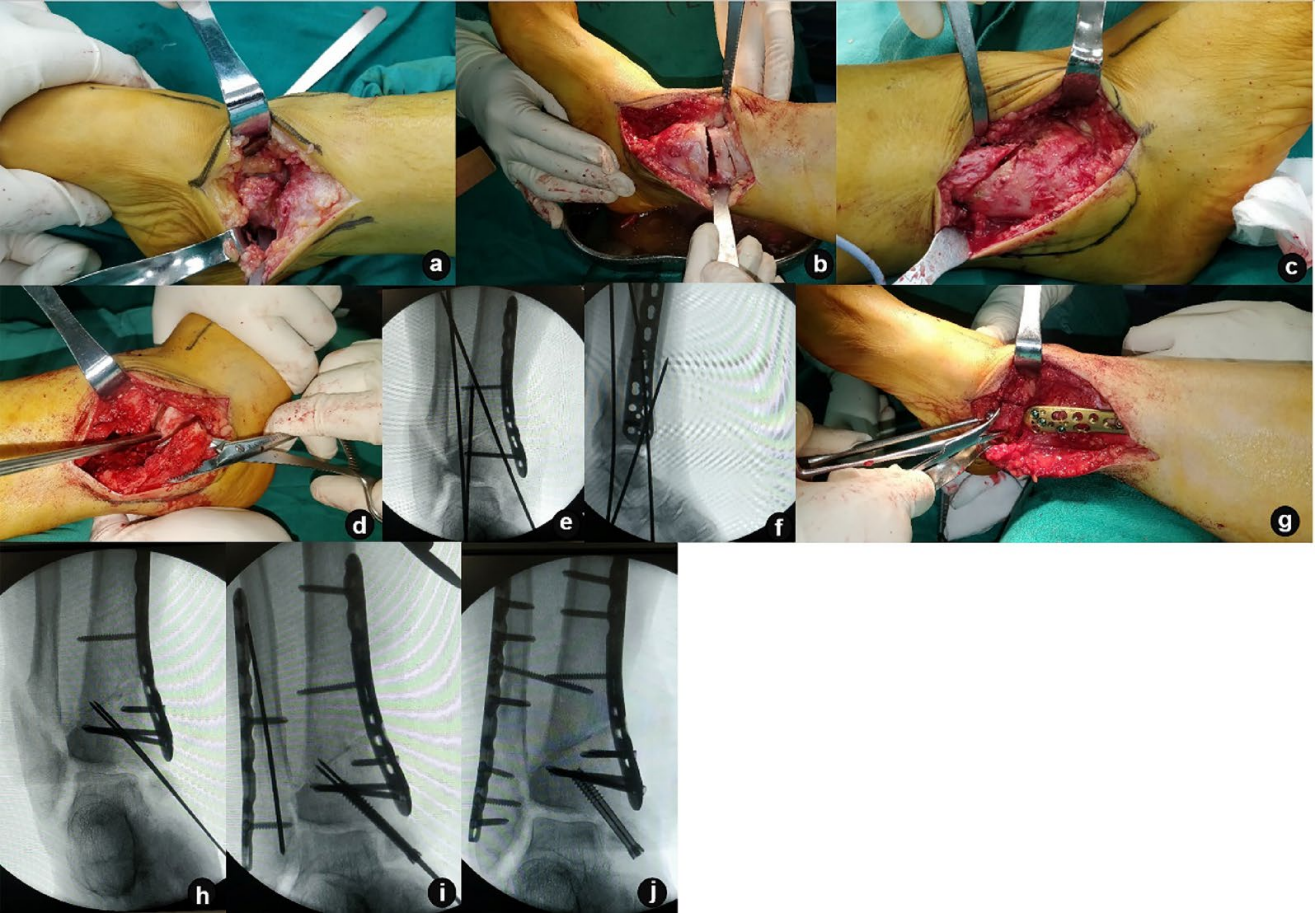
Intraoperative images of the case mentioned. a, b After the medial incision was made, the scar tissue around the ankle joint was exposed and cleaned, and a medial closed-wedge distal tibial osteotomy was conducted; c, d A lateral incision was made to verify that fibular fracture site was healed, a fibular osteotomy was conducted proximally to the original fracture line; e, f After the tibia was fixed with a medial tibial plate, the position was confirmed with satisfaction through anteroposterior and lateral X-rays fluoroscopy; g, h Reduction and fixation of old fractures of the medial malleolus was conducted with guide pin fixation; i, j the position was confirmed by fluoroscopy again after medial malleolar screws and plates for lateral malleolar fixation were placed
